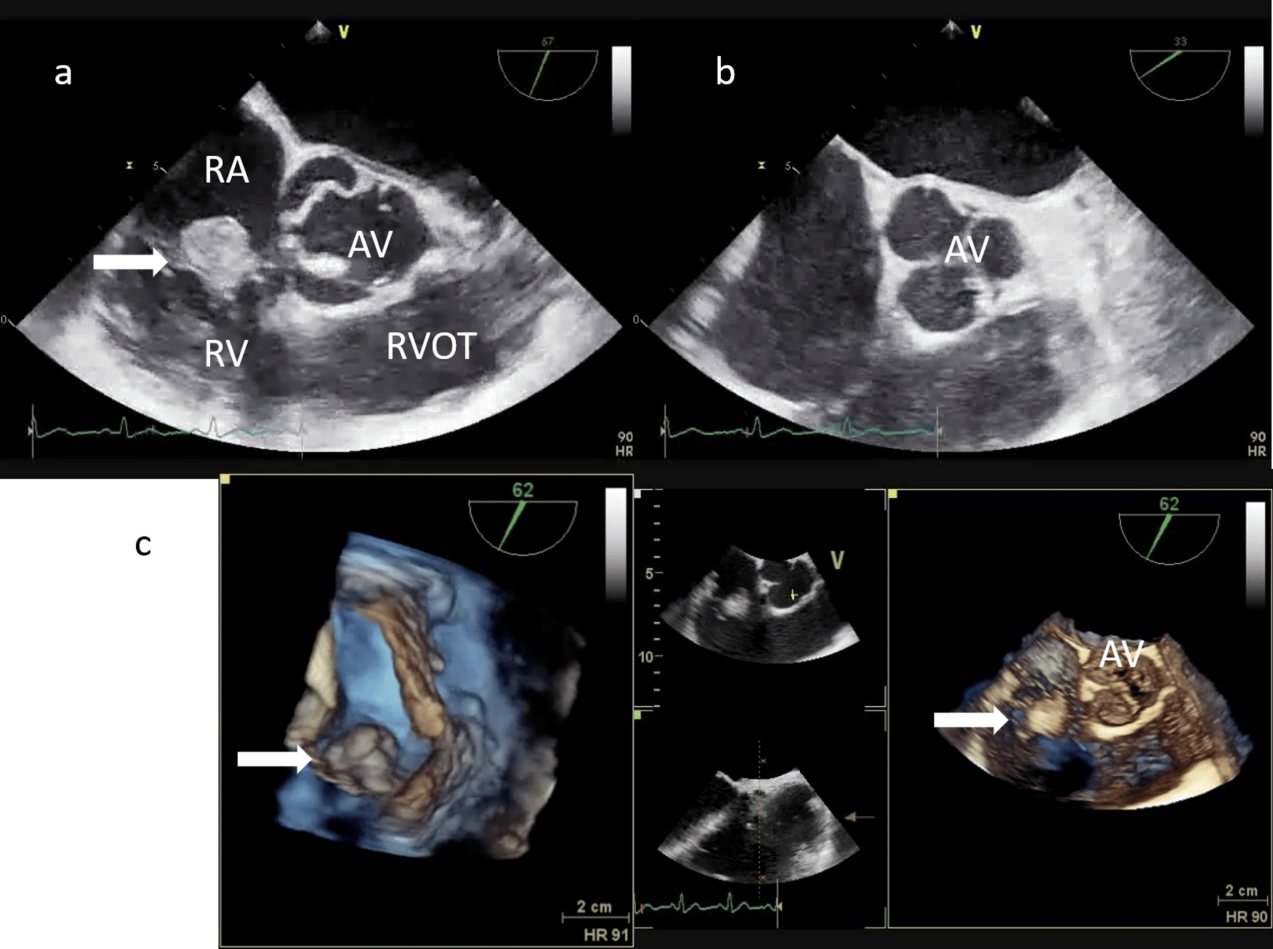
TEE (a to c) a: Arrow demonstrates the vegetation between the anterior tricuspid leaflet and the septal tricuspid leaflet during ventricular systole. b. Vegetation is not seen on the aortic valve. c: Three-dimensional TEE shows the vegetation between the anterior tricuspid leaflet and the septal tricuspid leaflet during ventricular systole. TEE: transesophageal echocardiography, AV: aortic valve, AL: anterior tricuspid leaflet, PL: posterior tricuspid leaflet, SL: septal tricuspid leaflet
